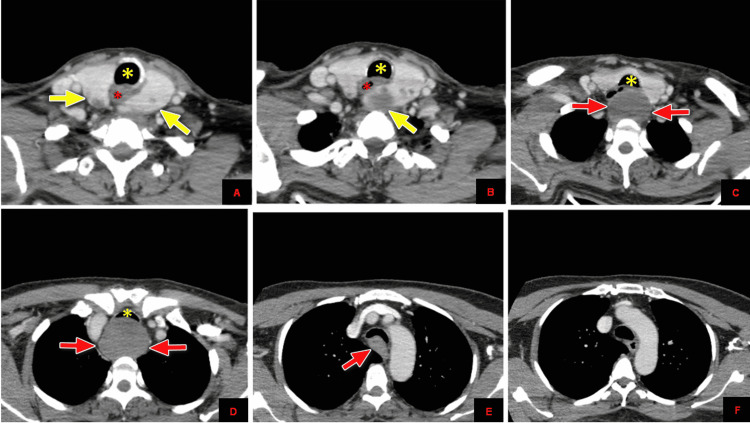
Contrast-enhanced CT scan of the chest with yellow arrows demonstrating that both thyroid glands are enlarged, showing multiple subcentimeter hypoattenuating nodules (A and B). A large exophytic oval-shaped solid lesion is seen arising from the posteroinferior pole of the left thyroid gland (red arrows) in C, which is extending in prevertebral space and markedly compressing and displacing the esophagus (red asterisk) and trachea (yellow asterisk) in D, E and F. It is smoothly marginated with no definite invasion.
